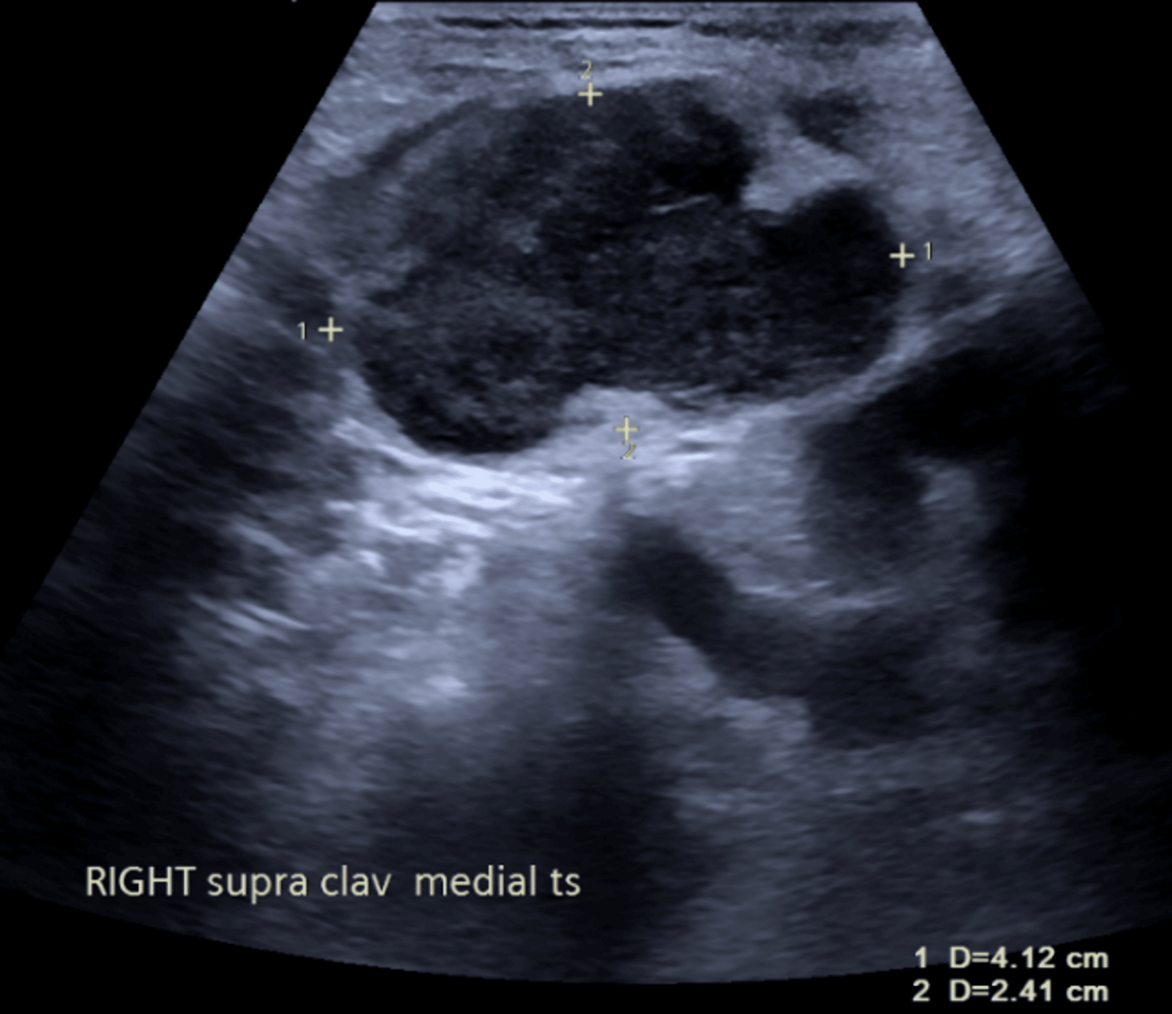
USS neck demonstrating lesion measuring 4.1 x 2.4 x 4.0 cm USS: Ultrasound scan.
